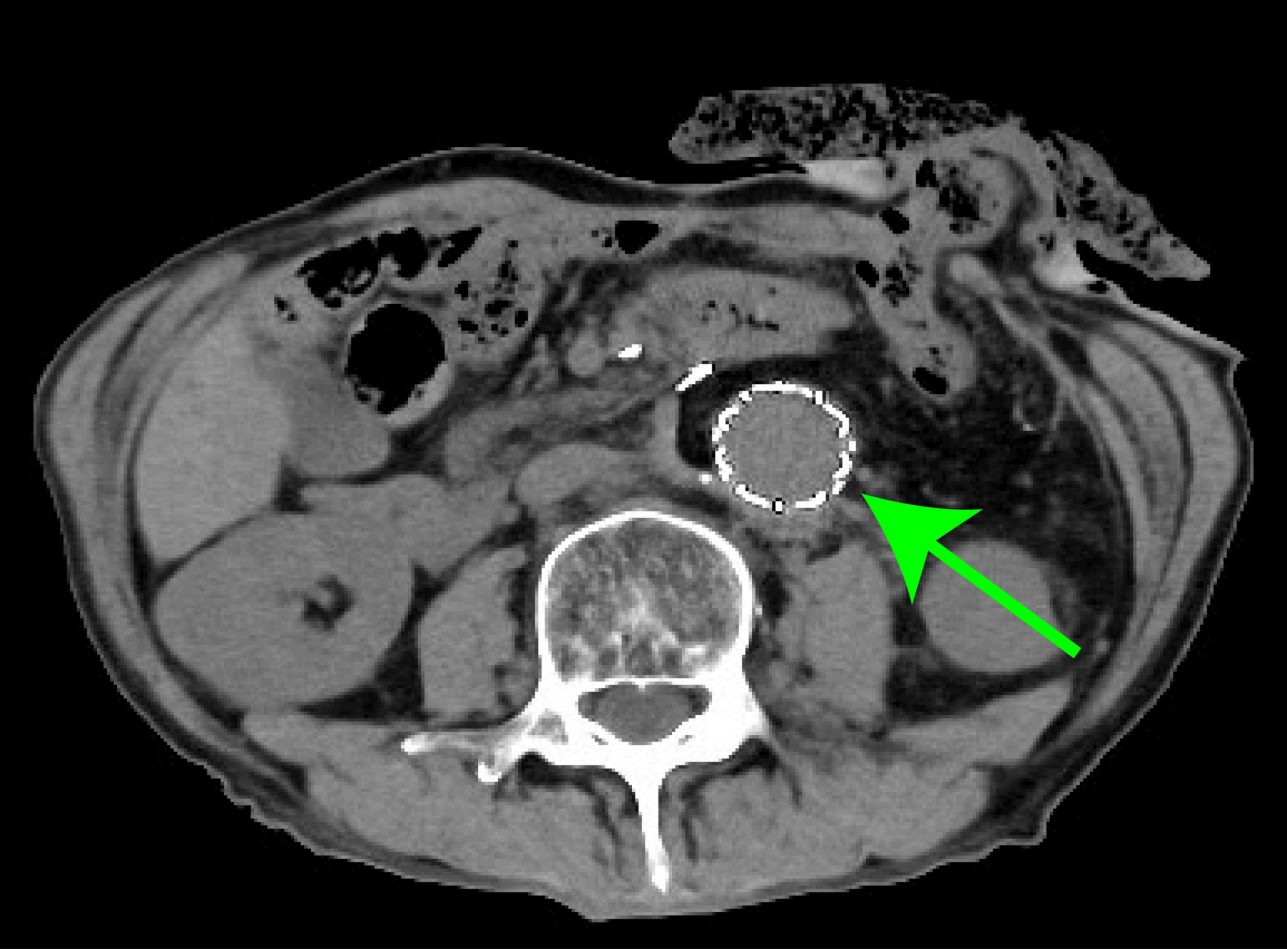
CT image at five-years post-operatively A stent graft was observed to be inserted into the artificial blood vessel. Surrounding the graft, the omentum was packed to provide reinforcement and promote infection control.
